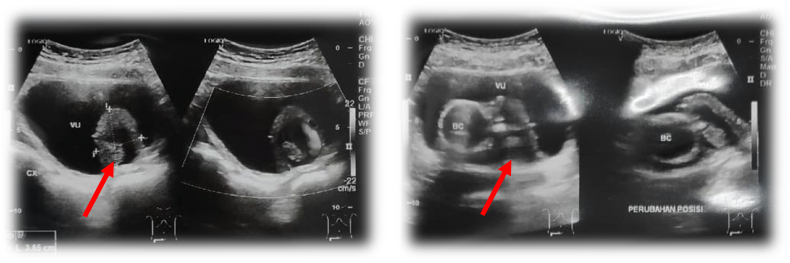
Abdominal ultrasound in patients showing hetero-echoic lesions contained dominant solid mixed cystic with calcification component in it, the size of the mass was 3.6 × 3.8 × 3.1 in the posteroinferior bladder wall (shown by red arrow). (For interpretation of the references to colour in this figure legend, the reader is referred to the web version of this article.)
